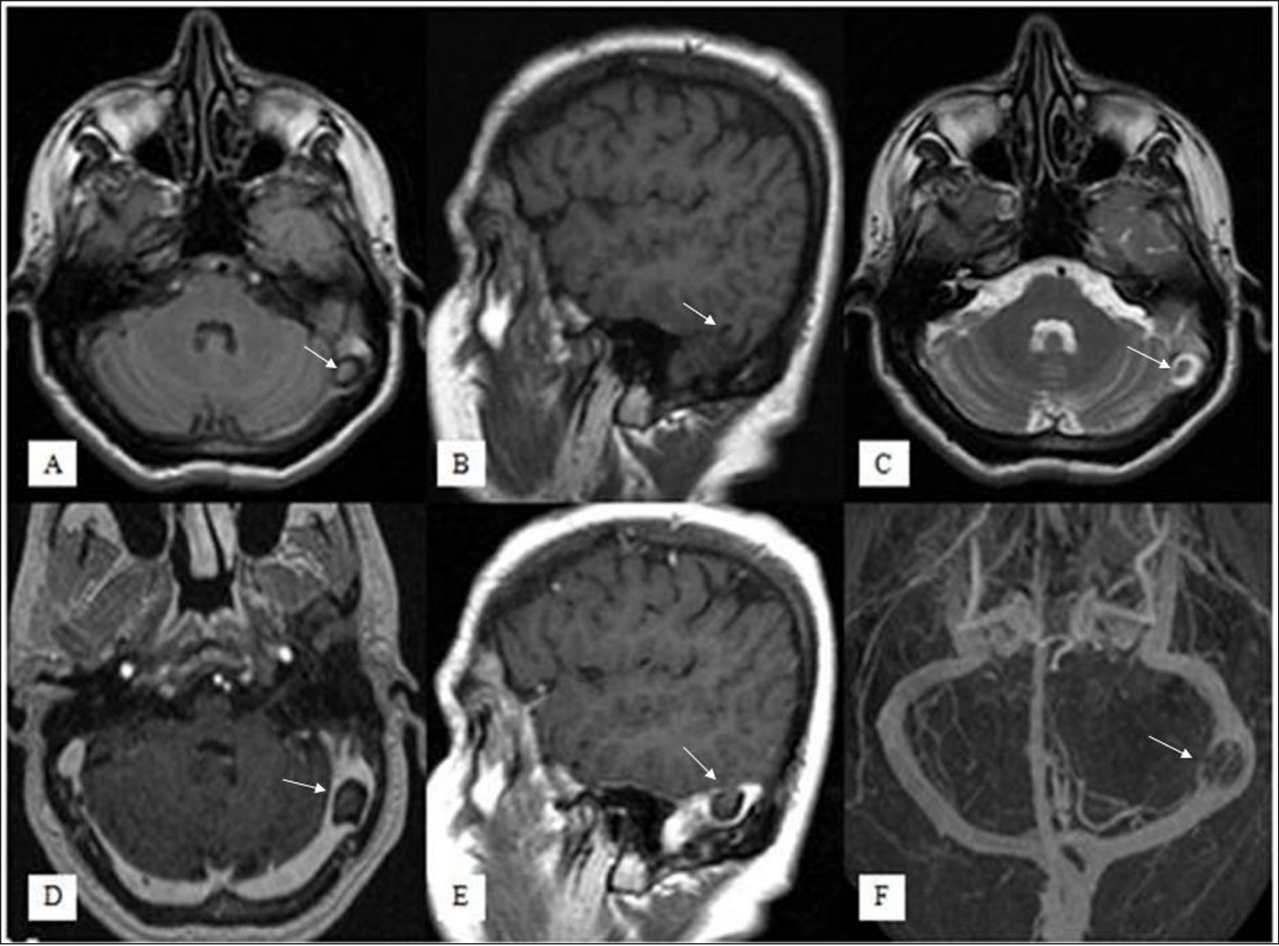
Fluid Attenuated Inversion Recovery axial (A), T1-weighted sagittal (B), T2-weighted axial (C), contrast-enhanced T1-weighted axial (D) and sagittal (E) images show a small herniation of temporal lobe parenchyma with surrounding CSF into left transverse sinus, that was isointense to brain parenchyma on all sequences (arrows). No pathological enhancement is seen but the brain herniation sac is causing moderate stenosis in the left transverse sinus. On venography imaging (F), there was left transverse sinus stenosis but no venous thrombosis.
